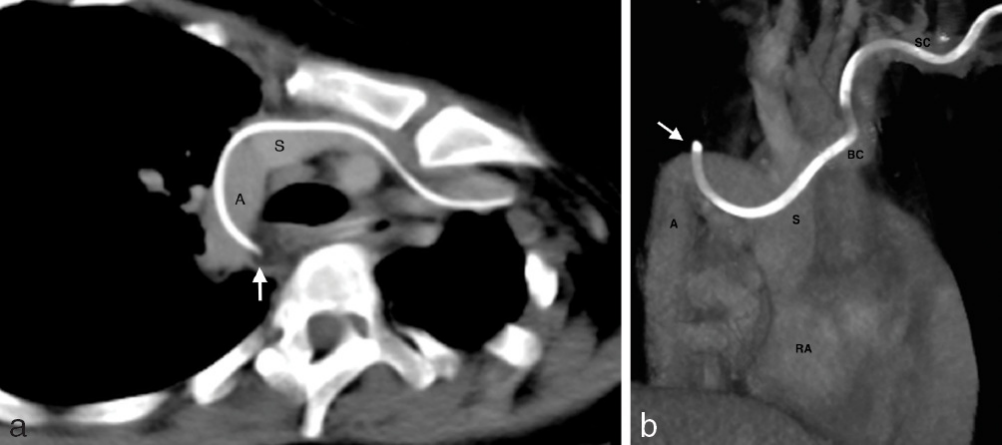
(a) Oblique axial slice from the CT scan obtained after drainage of the effusions shows that the PICC tip (white arrow) has perforated the wall of the azygos (A). The azygos is dilated and has a similar diameter to the SVC (S). (b) Coronal three-dimensional reconstruction also demonstrating perforation of the azygos (A) by the PICC (white arrow). There is variant drainage of the azygos into the right lateral wall of the SVC (S). This creates a favourable angle for migration of a left-sided PICC into the azygos. BC, left brachiocephalic vein; PICC, peripherally inserted central venous catheter; RA, right atrium; SC. left subclavian vein; SVC, superior vena cava.
